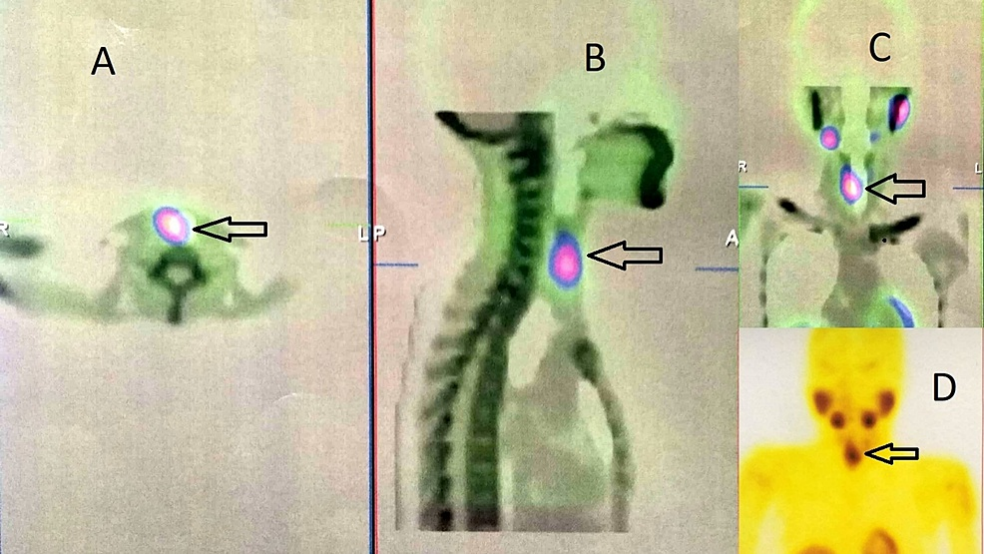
Transaxial (A), sagittal (B), and coronal (C) SPECT-CT of parathyroid glands showing (arrows) focal area of increased radiotracer concentration in the right side of the neck at the level of C5-C7 vertebra suggestive of parathyroid adenoma or carcinoma. Delayed static image of Tc99m sestamibi parathyroid scintigraphy (D) showing (arrow) persistent focal activity in the abovementioned area. SPECT-CT: single-photon emission computed tomography-computed tomography
